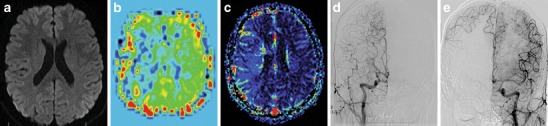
A 34-year-old male with a history of two transient ischemic attacks and normal neurological examination. Standard MRI was normal. a DWI (*b* = 1,000). b PASL sequence shows hypoperfusion in the distribution of the right MCA. c Contrast perfusion is concordant with PASL. DSA shows obliteration of the right MCA with very good collateral flow from the d ipsilateral and also e contralateral vascular territories. DWI, diffusion-weighted imaging; PASL, pulsed arterial spin labeling; MCA, middle cerebral artery; DSA, digital subtraction angiography
